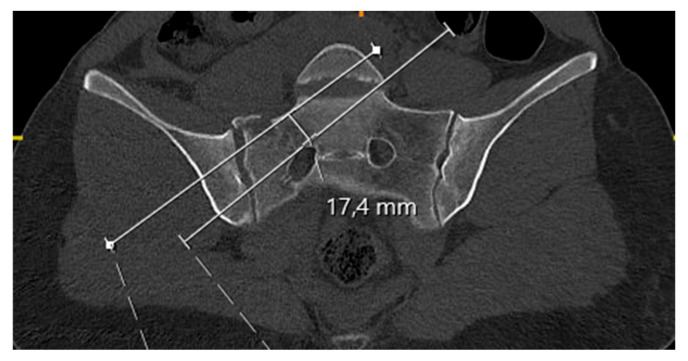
Preoperative measurement of the narrowest width of the S1 osseous corridor on coronal CT.
